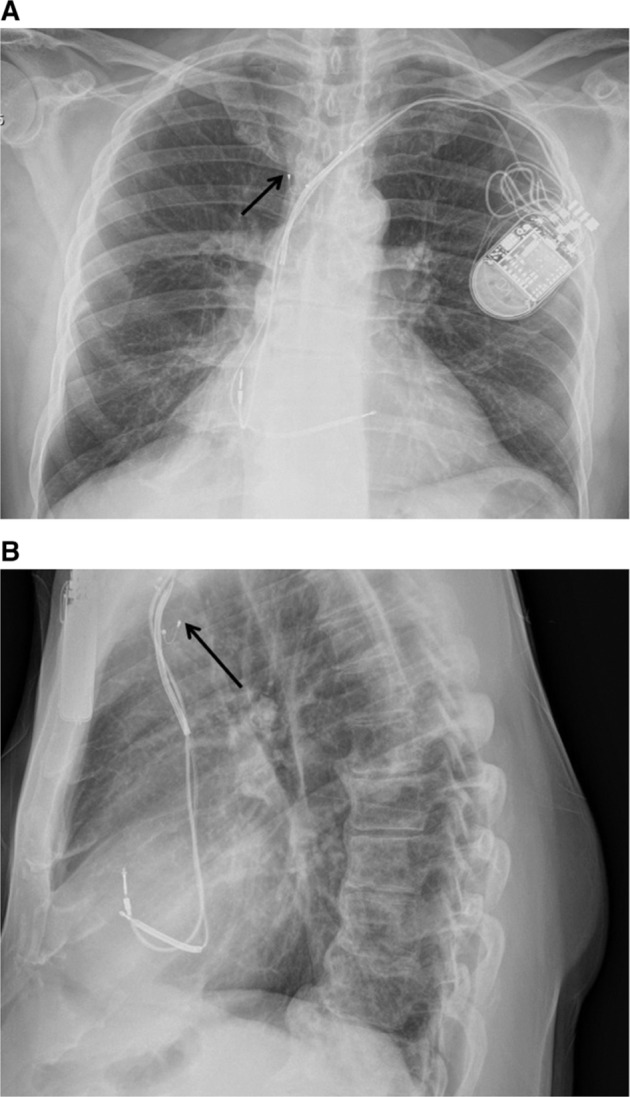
The arrows on the posteroanterior (A) and lateral (B) chest X-rays demonstrate retraction of the LV lead to the superior vena cava, resulting in phrenic nerve stimulation.
